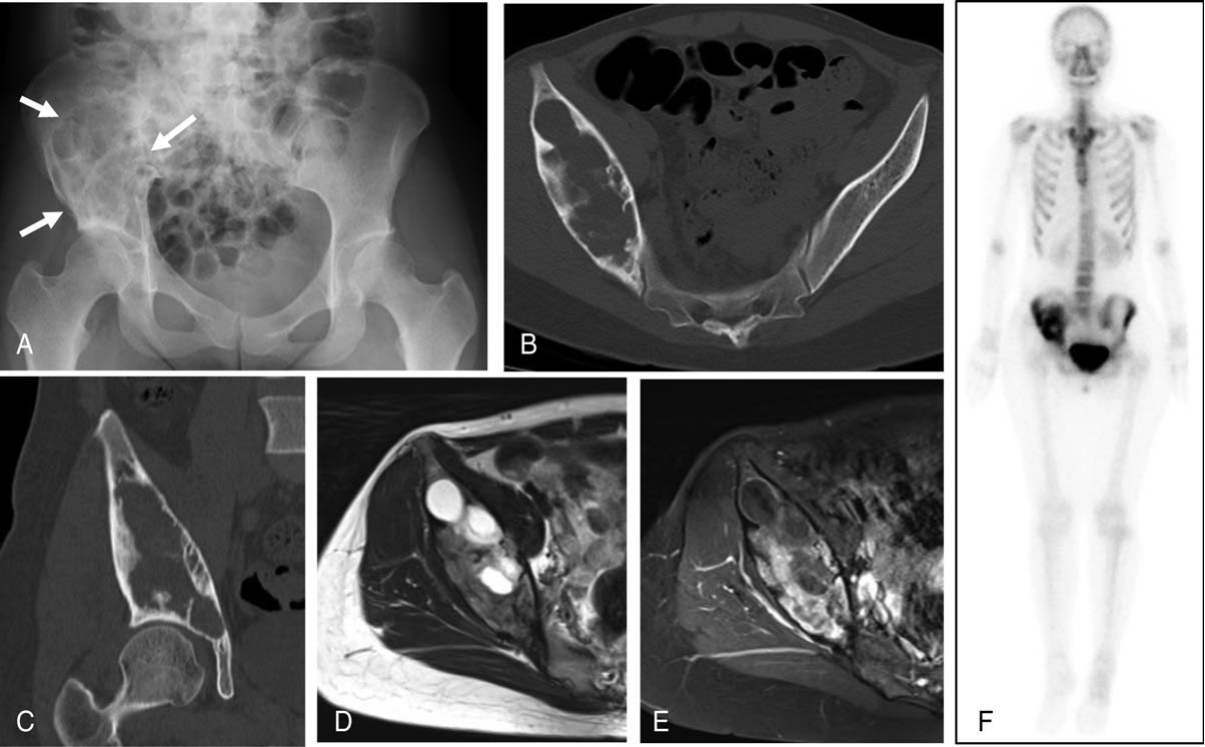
Images at initial visit (A) Plain radiograph showing a large osteolytic lesion (arrows) on the right ilium. (B) Axial view of pelvic computed tomography (CT) showing an expansile lytic lesion with cortical thinning. (C) Coronal CT showing the lesion extending to the subchondral bone of the acetabulum. (D) Axial T2-weighted magnetic resonance (MR) imaging revealed a multilocular lesion with high signal intensity, and (E) T1-fat suppressed axial MR image showing the lesion to be heterogeneously enhanced with gadolinium. (F) Bone scintigraphy showing increased focal uptake in the right ilium.
